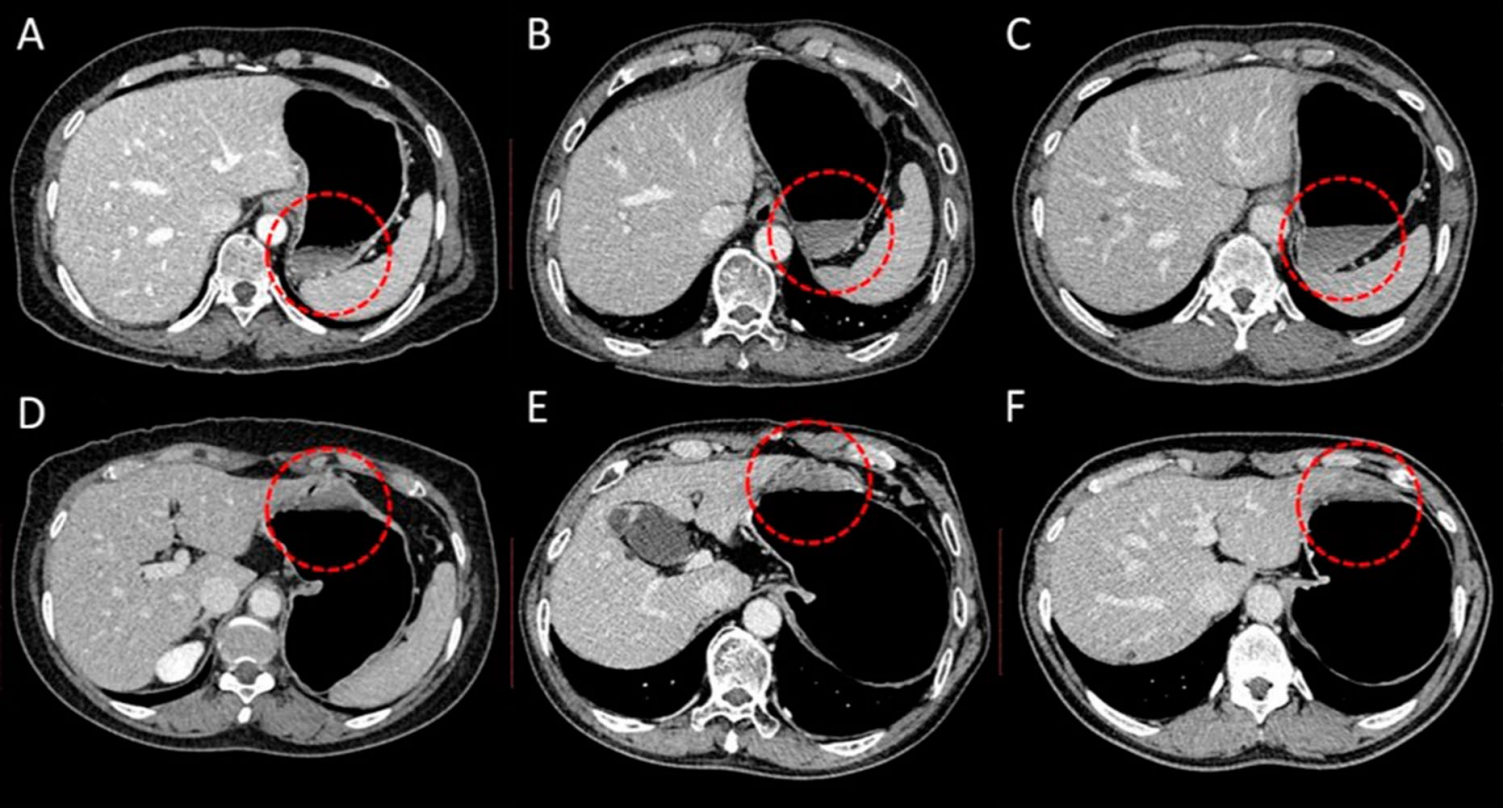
Three patients underwent computed tomographic gastrography (CTG) in the supine (A)–(C) and prone (D)–(F) positions. Each column represents one patient (A), (D): Patient 1; (B), (E): Patient 2; (C), (F): Patient 3. The residual fluid filling some portion of the gastric cavity depending on the scanning position is outlined in red.
